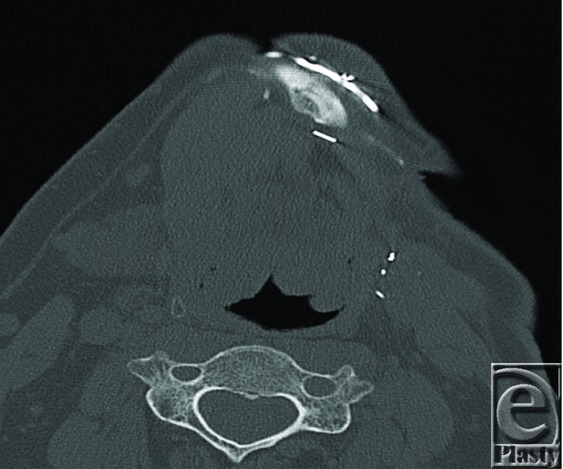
Computed tomographic scan showing problematic region of the plate.
